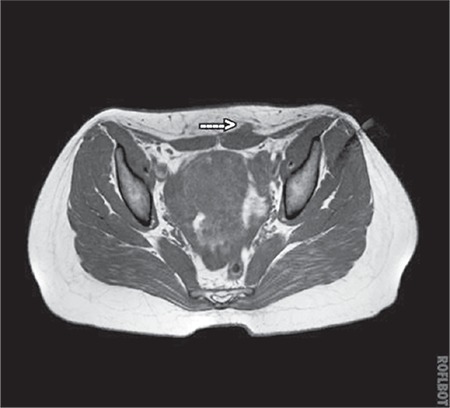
Magnetic resonance image axial
